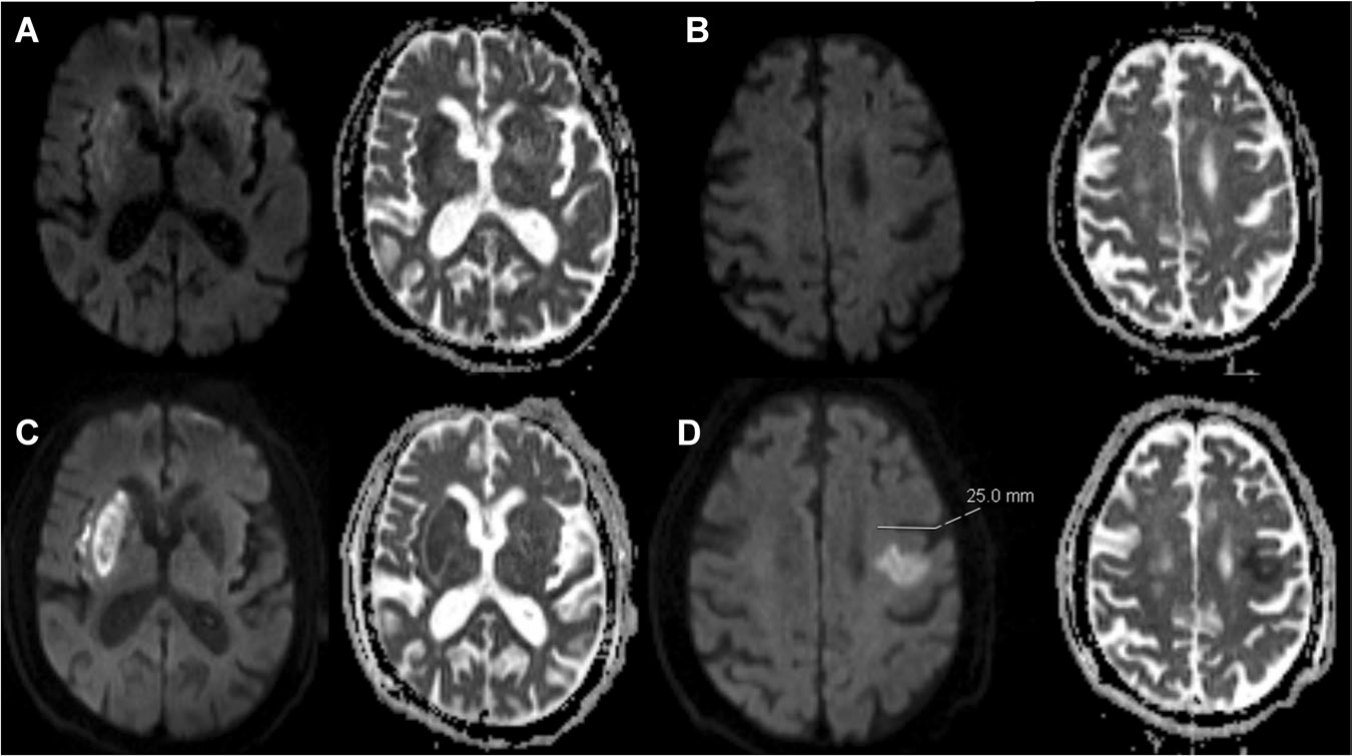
Large infarct in new territory without catheter manipulation across the ostium (type IIIB). (A,B) preinterventional diffusion-weighted imaging/apparent diffusion coefficient revealed mild diffusion restriction within the striatocapsular region and insular cortex on the right-hand side. No diffusion restriction in the contralateral hemisphere is delineable. (C,D) postinterventional DWI/ADC revealed marked diffusion restriction in the right middle cerebral artery territory without substantial infarct growth; however, a new DWI/ADC lesion is seen in the contralateral hemisphere in close proximity to the precentral sulcus, corresponding to a size type III (>20 mm), manipulation type B (the catheter was not manipulated past the ostium of the new territory) infarct in new territory.
